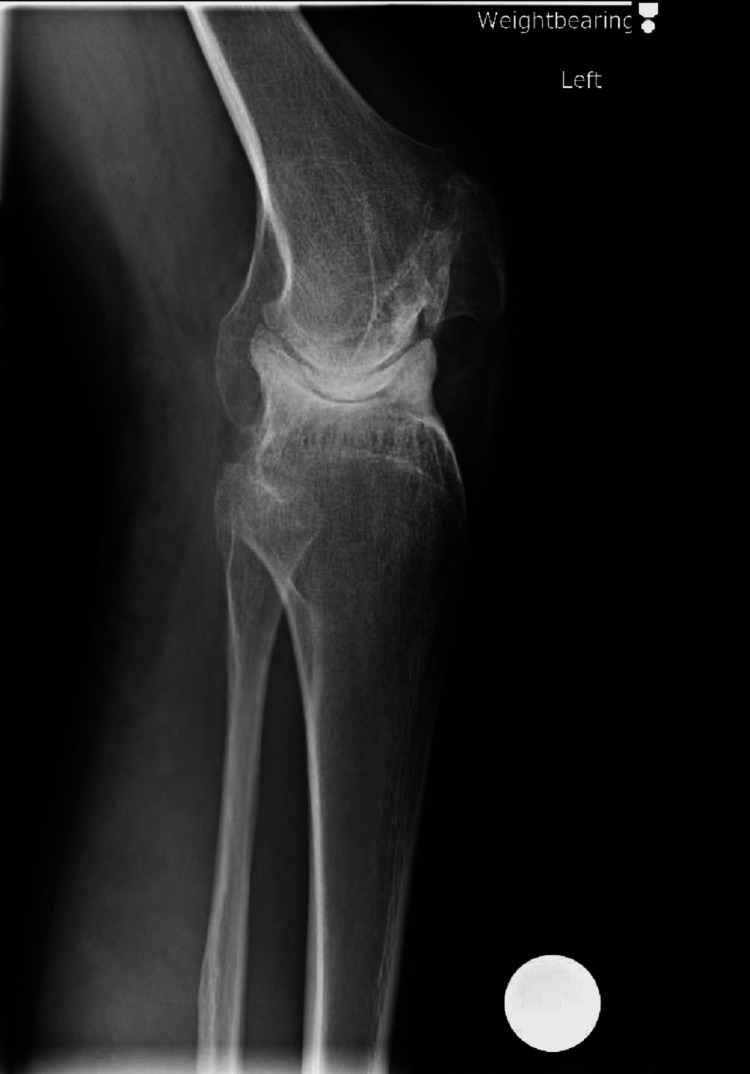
Preoperative lateral X-ray of the left knee showing concave deformity of the lateral tibial plateau.
